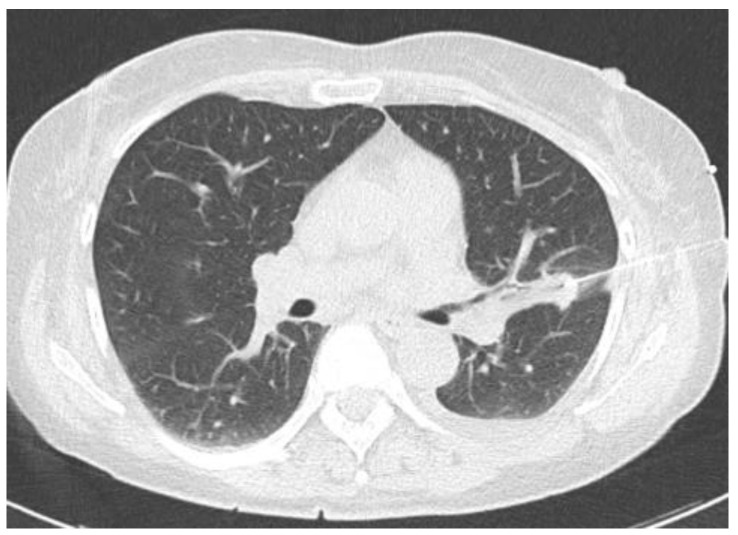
CT-guided injection of the [99mTc]-tilmanocept.
